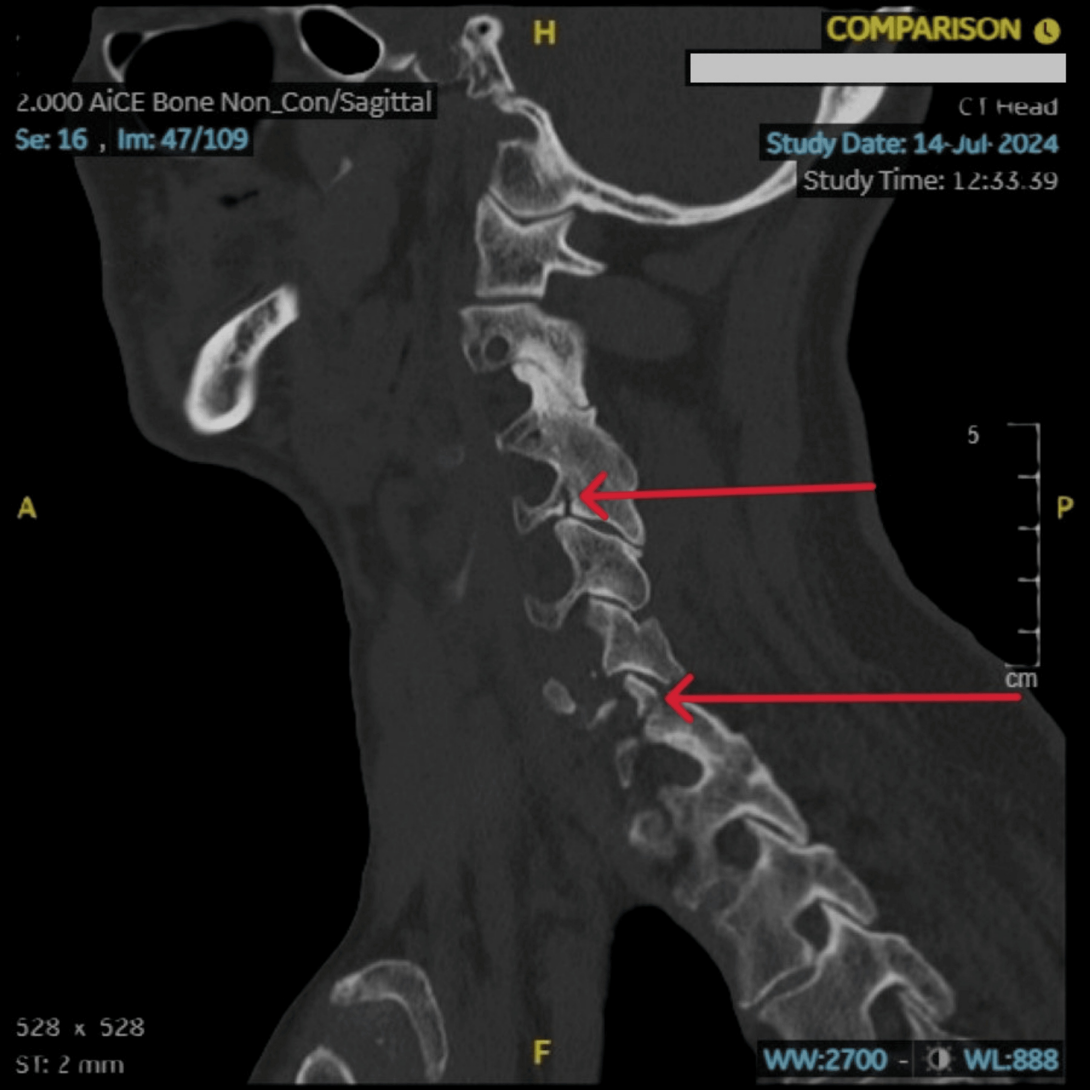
CT scan of head and cervical spine Minimally displaced fracture of the left foramen transversarium of the C4 vertebra and mildly displaced fracture of the left superior articular facet of the C7 vertebra, with minimal diastasis of the left-sided C6-C7 facet joint on a sagittal view of a non-contrast CT scan of the head and cervical spine. These findings are marked by a red arrow on the scan.
